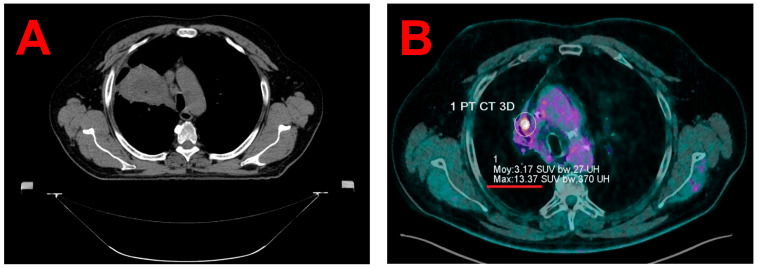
An axial view from planning CT of the initial 2020 radiotherapy treatment, showing the extensive infiltration of squamous cell carcinoma in the right upper lobe, (A) and an axial view from 7 December 2023 follow-up PET/CT, showing the progression of a residual para-mediastinal hypermetabolic focus located within a region of extensive radiation-induced fibrosis in the right upper lobe (SUVmax 13.4 vs. 4.7 in January 2023) (B).
